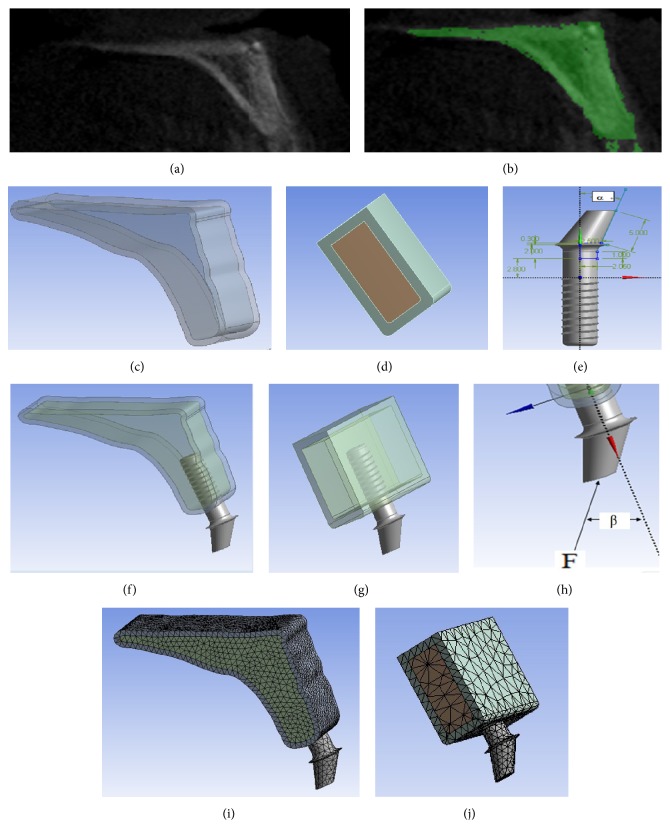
Simplified patient-specific models: A cone-beam computerized tomography scan projection of a maxillary central incisor region was obtained (a), the outline of the image was manually converted (b). Simplified patient-specific models were created by extruding the simplified cross-sectional image (c). Simplified models were used in this study (d). The geometry of the implant-abutment complex was developed based on the models described in the previous study;  *α* was abutment angulation (e). All models were combined by Boolean operations (f, g). (h)* F*: occlusal force;  *β* (loading angle) = 0°, 15°, and 20°. (i, j) Meshed implants and bone.
